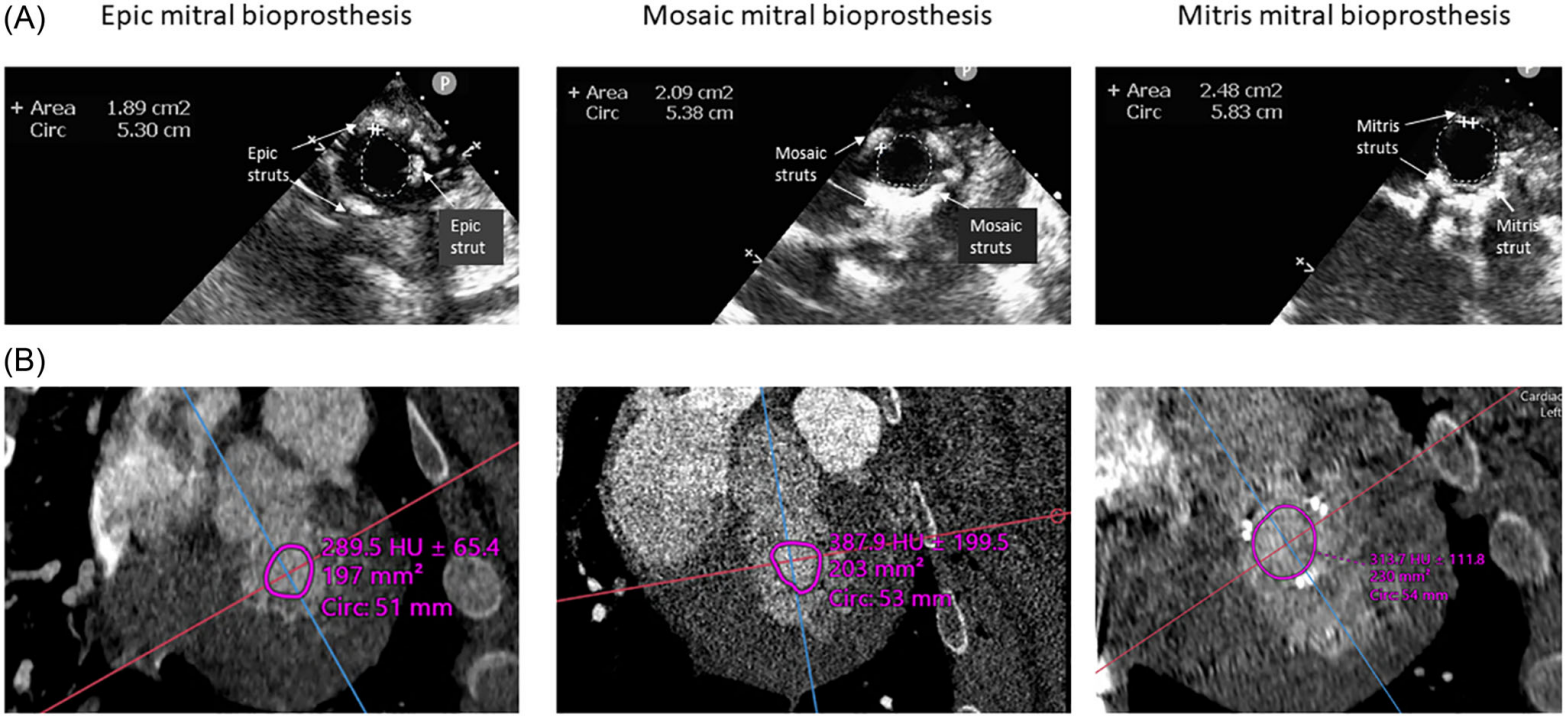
Maximal surgical valve opening area of mitral bioprostheses' leaflets. (A) shows the epicardial 2D echocardiographic planimetry measurement of the surgical mitral bioprosthesis leaflet tips at maximal mid‐end diastolic opening by type of bioprosthesis with trend in ascending order, 27‐mm Epic with the smallest surgical valve opening area, followed by the 27‐mm Mosaic and largest in the 25‐mm Mitris. (B) demonstrates the corresponding maximal mid‐end diastolic mitral bioprosthesis surgical valve opening area leaflet tip planimetry by multiplanar 3D‐CT evaluation with similar trends. CT, computed tomography
